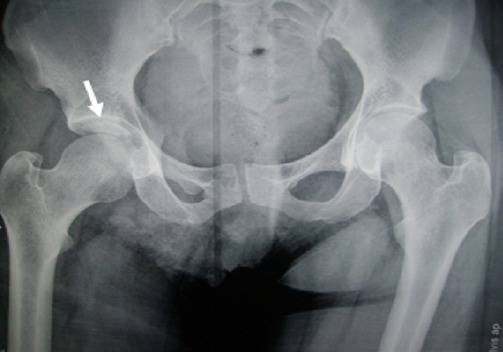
Radiographie du bassin de face objectivant une fracture pipkin II de la tête fémorale indentée sur le rebord postérieur du cotyle, le fragment céphalique est déplacé en polaire supérieur
